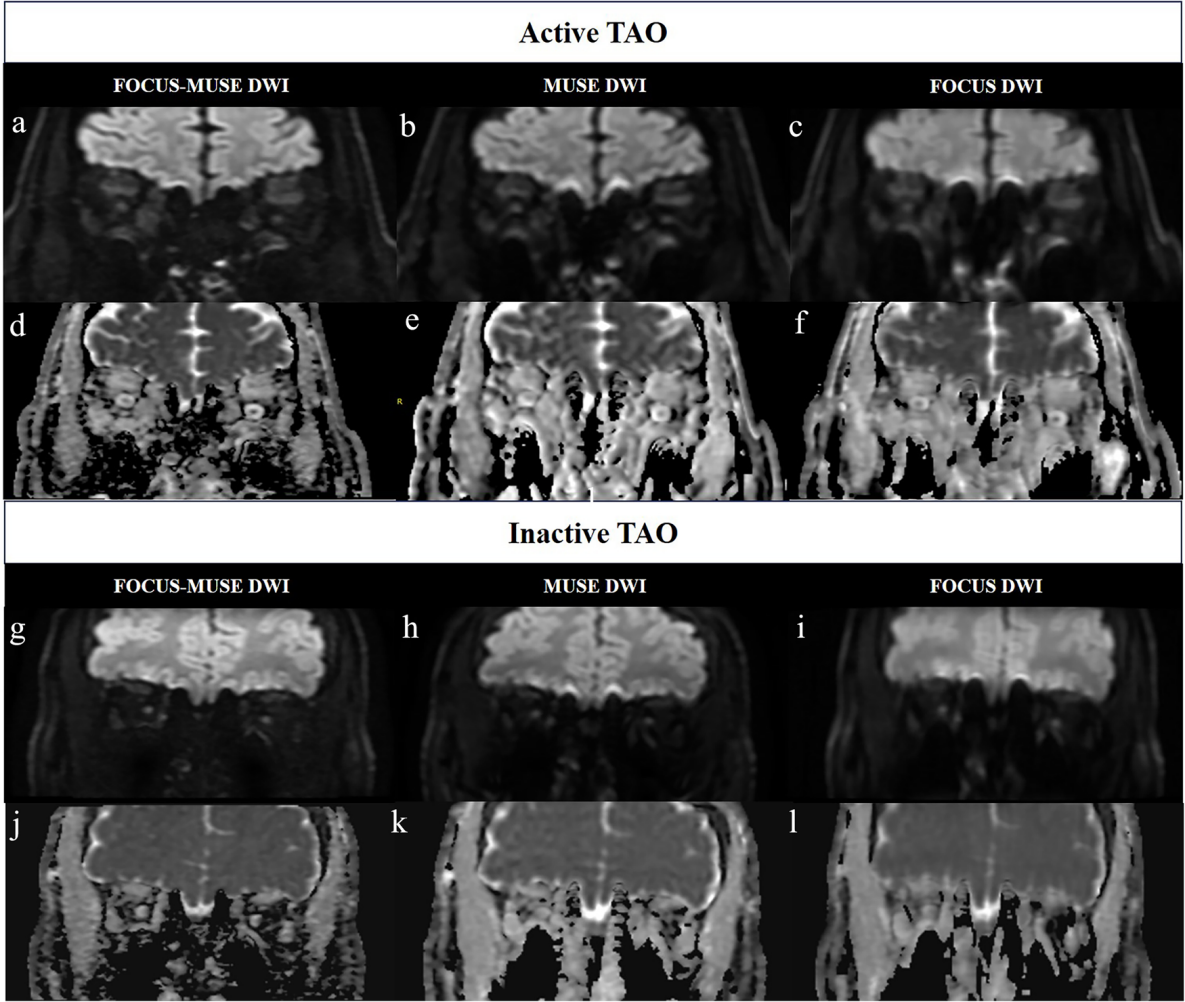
Representative images with FOCUS-MUSE DWI (a, g; *b* = 1000 s/mm^2^), MUSE DWI (b, h; *b* = 1000 s/mm^2^), FOCUS DWI (c, i; *b* = 1000 s/mm^2^) and corresponding ADC images (d, j; e, k; f, l) of an active (male, 71-year-old, CAS = 5) and an inactive (male, 36-year-old, CAS = 2) patients with TAO, respectively. FOCUS-MUSE DWI showed fewer artifacts and geometric distortion, superior image quality, and enhanced sharpness of boundaries compared to MUSE DWI and FOCUS DWI. For active TAO patients, the mean nADC values of left and right eyes were 1.26 and 1.14, respectively. For an inactive TAO patient, the mean nADC values of the left and right eyes were 0.90 and 0.97, respectively. DWI, diffusion-weighted imaging; ADC, apparent diffusion coefficient; TAO, thyroid-associated ophthalmopathy. nADC, normalized apparent diffusion coefficient.
